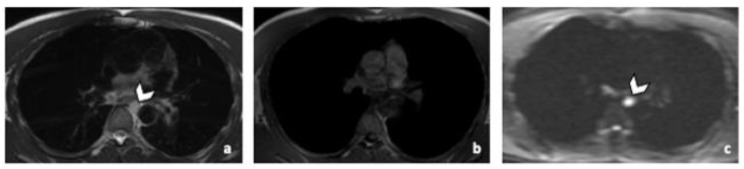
Comparison of WB sequences in a 39-year-old patient with NSGCC. Axial T2-weighted (a), 3D T1 GRE In Phase (b) and high b-value DWI (b = 1000 s/mm^2^) MR images. One of the readers missed an enlarged paraoesophageal lymph node (arrowhead) demonstrating a high signal intensity in T2 (a), barely visible in T1 and presenting a restricted Diffusion (c). Abbreviations: NSGCC: Non-Seminomatous Germ Cell Cancer, 3D: Three Dimensional, T1 GRE: T1 Gradient Echo, DWI: Diffusion Weighted Imaging.
